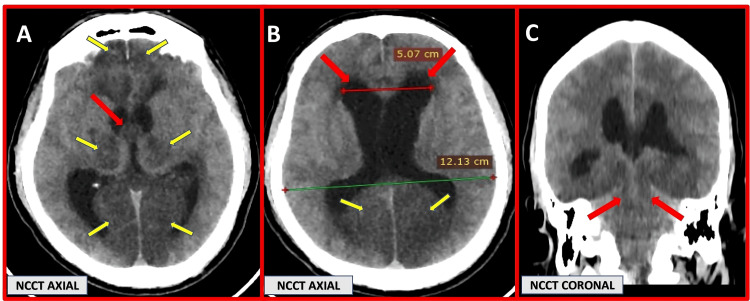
NCCT brain images: (A and B) axial sections and (C) coronal reformatted image (A) Axial NCCT shows a hypodense cystic lesion at the roof of the third ventricle (red arrow), measuring approximately 13 × 11 mm (HU: 12), obstructing the foramen of Monro. Acute infarcts are noted in the bilateral thalami and frontoparietal lobes (yellow arrows). (B) Axial CT image demonstrates marked dilatation of the lateral ventricles (Evans index: 0.4) with additional acute infarcts in the bilateral parietal lobes (yellow arrow). (C) Coronal CT section reveals central transtentorial herniation (red arrow) and diffuse cerebral edema. Note: All images are original and obtained from the institutional study dataset. NCCT, non-contrast CT
